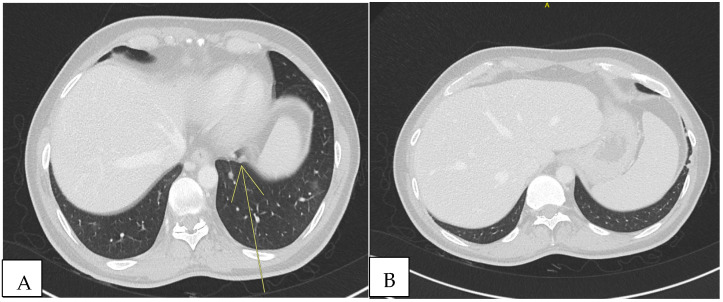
Total-body CT scan revealed non-specific pulmonary consolidations. Other diseases could explain these observations, such as sarcoidosis, neoplasm, or other infectious diseases (TB, NTM, herpesviruses, cryptococcosis). (A) Nodule in retrocardiac area (yellow arrow). (B) Two nodules in subpleural area.
